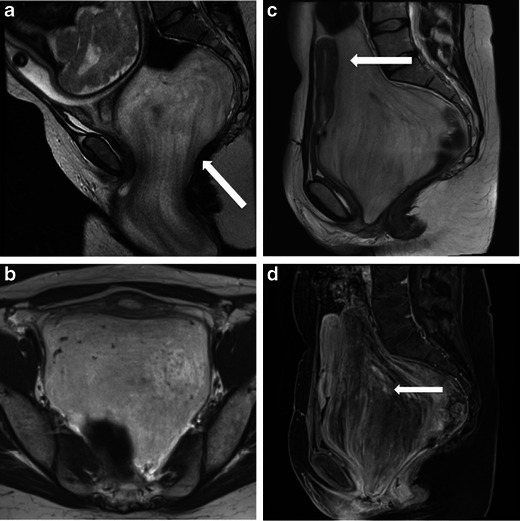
Imaging of aggressive angiomyxoma. a A 38-year-old woman presented with abdominal pain during pregnancy. Sagittal T2-weighted MRI image shows a large pelvic mass (*arrow*), which is predominantly T2 hyperintense with a ‘whorled’ appearance. b, c Follow-up imaging post-partum showed interval growth of the mass. The mass is displacing structures, the uterus can be seen displaced anteriorly (*arrow*). d Sagittal T1-weighted image post gadolinium shows the characteristic swirling pattern of contrast enhancement, typical of aggressive angiomyxoma (*arrow*)
